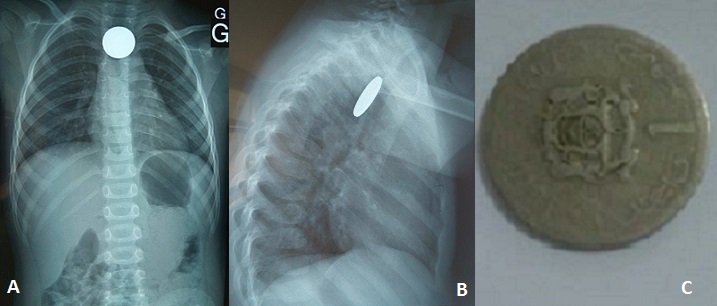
(A) radiographie thoracique de face qui montre un énorme objet radio-opaque se projetant au niveau de la région cervicale; (B) cliché de profil qui confirme la position postérieure du CE œsophagien par rapport aux clartés antérieures du larynx, de la trachée et de la carène; (C) la pièce de monnaie après extraction sous bronchoscopie rigide
